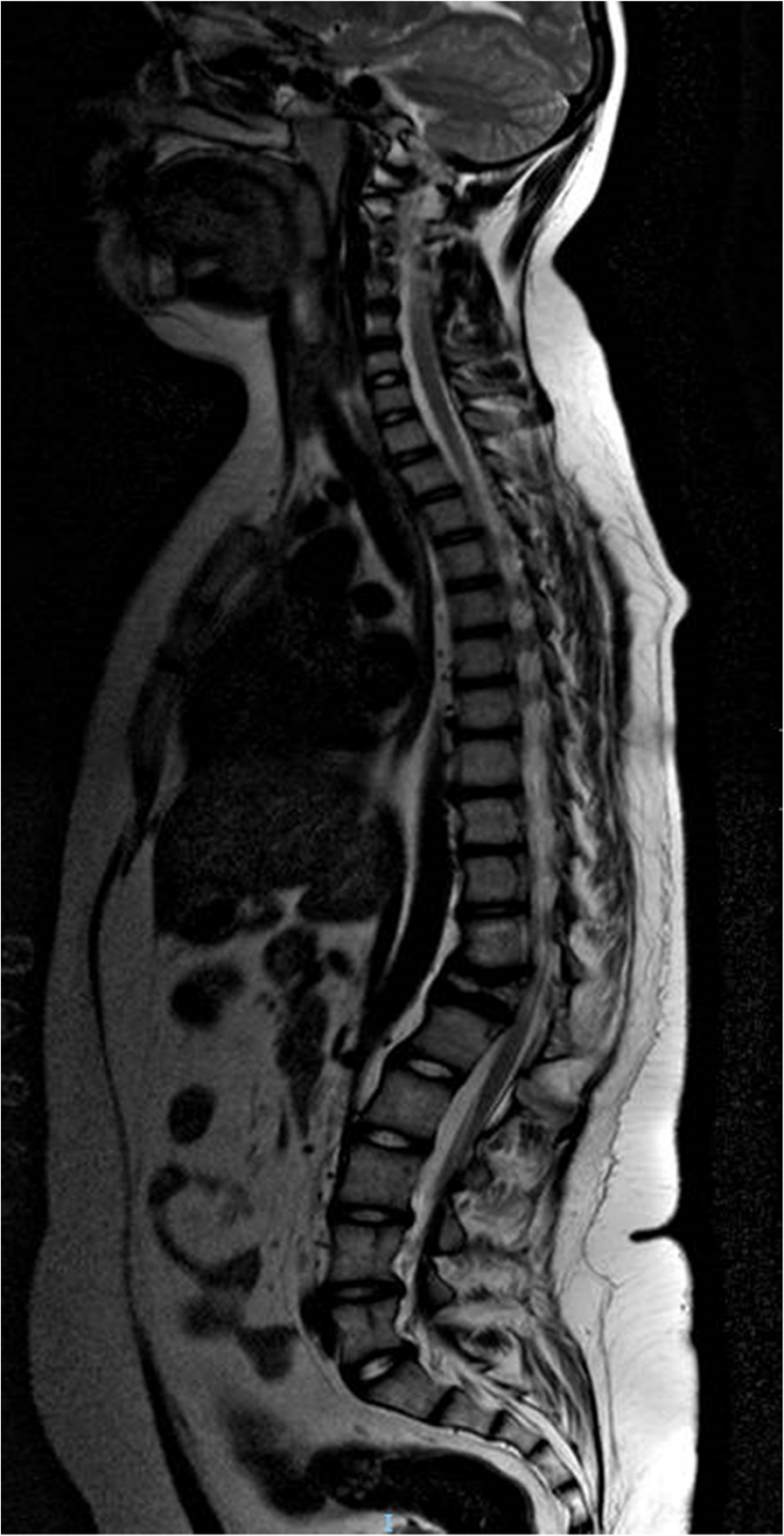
Follow-up magnetic resonance imaging of the spine. Magnetic resonance imaging of the spine, T2-weighted sagittal plan, a year after completion of treatment: residual vertebral collapse at Th12 with finding of “vertebra plana”; no residual tumor mass
